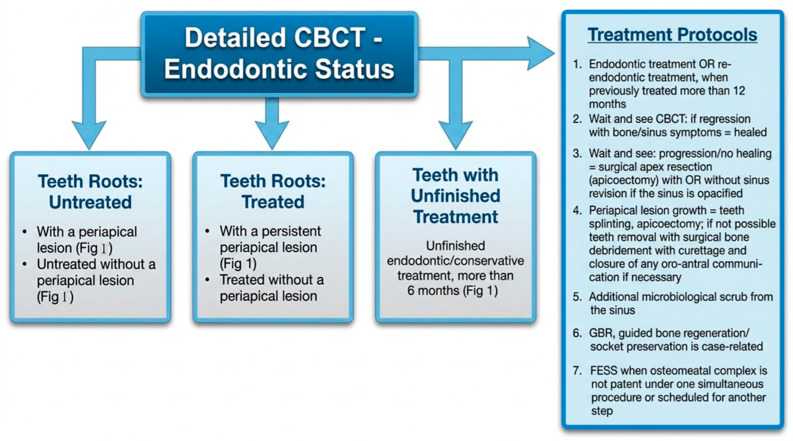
Proposed protocol based on CBCT images on endodontic-related factors influencing ODS.
